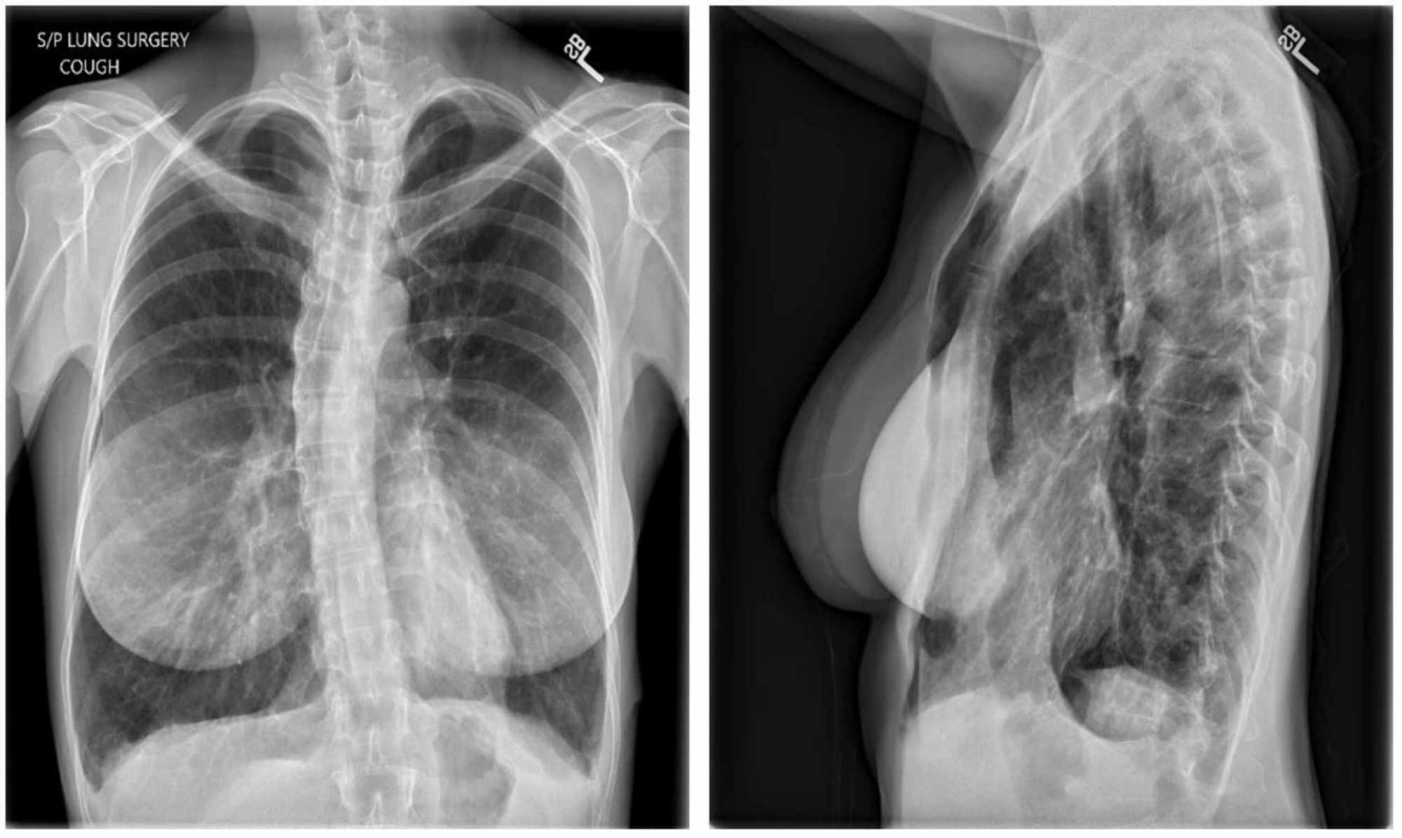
X-ray chest PA and lateral post-surgery No acute cardiopulmonary abnormality identified; chronic lung changes that are consistent with lymphangioleiomyomatosis PA: posteroanterior
